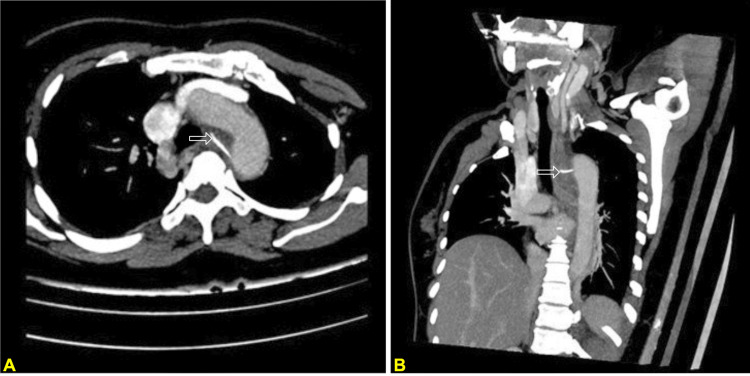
CT scan demonstrated a linear radiopaque foreign body (#2.3 cm), consistent with a fish bone (white arrows) CT: computed tomography The object was located at the level of the T3 vertebra, and the posterior end pointed toward the descending aorta. Associated esophageal wall thickening and periesophageal edema were noted. No definite abscess or pneumomediastinum was demonstrated. (A) Axial plane, (B) coronal plane. White arrows (A and B panels) demonstrated the fish bone
